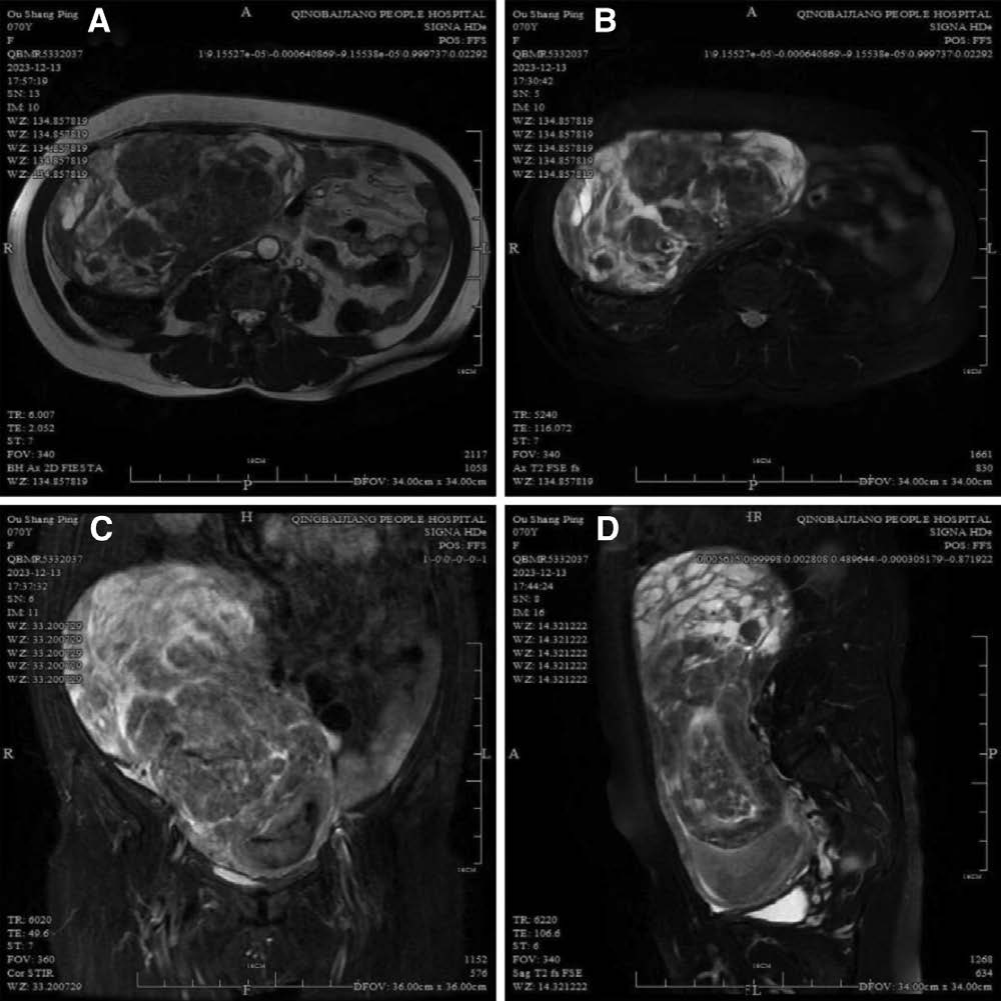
MRI imaging of a giant tumor on the right side of the abdominopelvic cavity, with some areas of high signal, which is possibly a neoplastic lesion of uterine origin or uterine sarcoma. (A) Cross-sectional image of T1-weighted image. (B) Cross-sectional image of T2. (C) Coronary image of T2. (D) Sagittal image of T2. MRI = magnetic resonance imaging.
